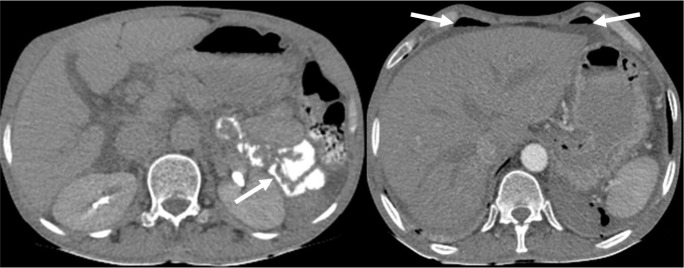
Oral and intravenous contrast abdominal computed tomography scan. The leak of the contrast material from gastrointestinal tract and its extension to the left pararenal space was seen. Ascites and pneumoperitoneum were seen in subhepatic space
